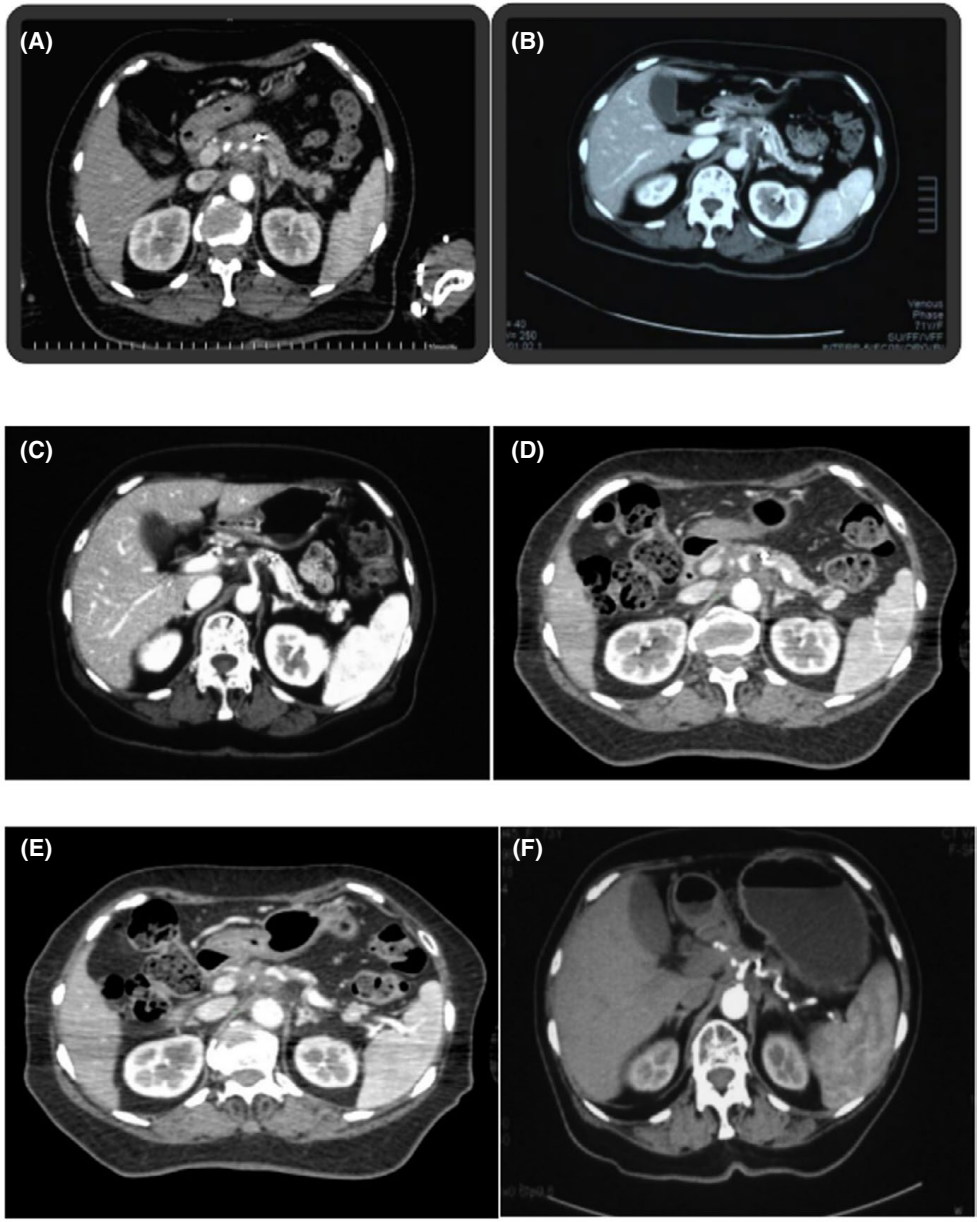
A. CT scan prior to the first‐course treatment shows a mass in the pancreatic head. B, C. CT scan 3 months and 9 months following the first SBRT course. D. CT scan prior to the second‐course treatment shows the local recurrence of the tumor in the pancreatic head. E. CT scan 1 months following the second SBRT course. F. CT scan 6 months following the second SBRT course
